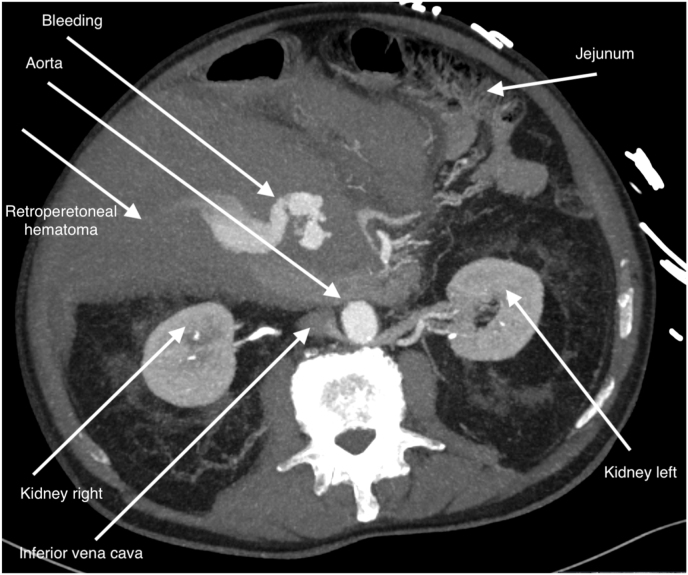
Transversal CT image: in a short period, a peritoneal hematoma develop with active leakage.
